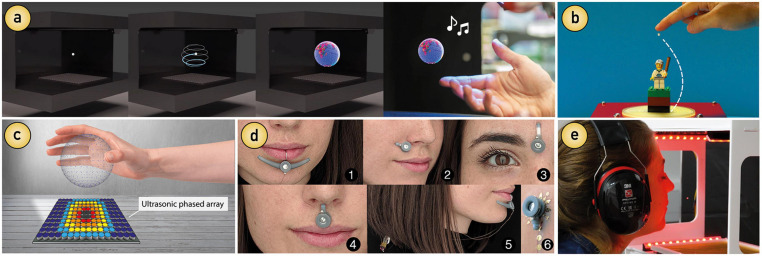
Emerging multisensory technologies exemplifying interface and device advancements. (a) Particle-based volumetric displays (Hirayama et al., 2019). (b) Acoustic metamaterials that bend the sound (Norasikin et al., 2018). (c) Mid-air haptic 3D shapes produced by focused ultrasound (Carter et al., 2013). (d) On-face olfactory interfaces (Wang et al., 2020). (e) Gustatory experiences based on levitated food (Vi et al., 2020).
